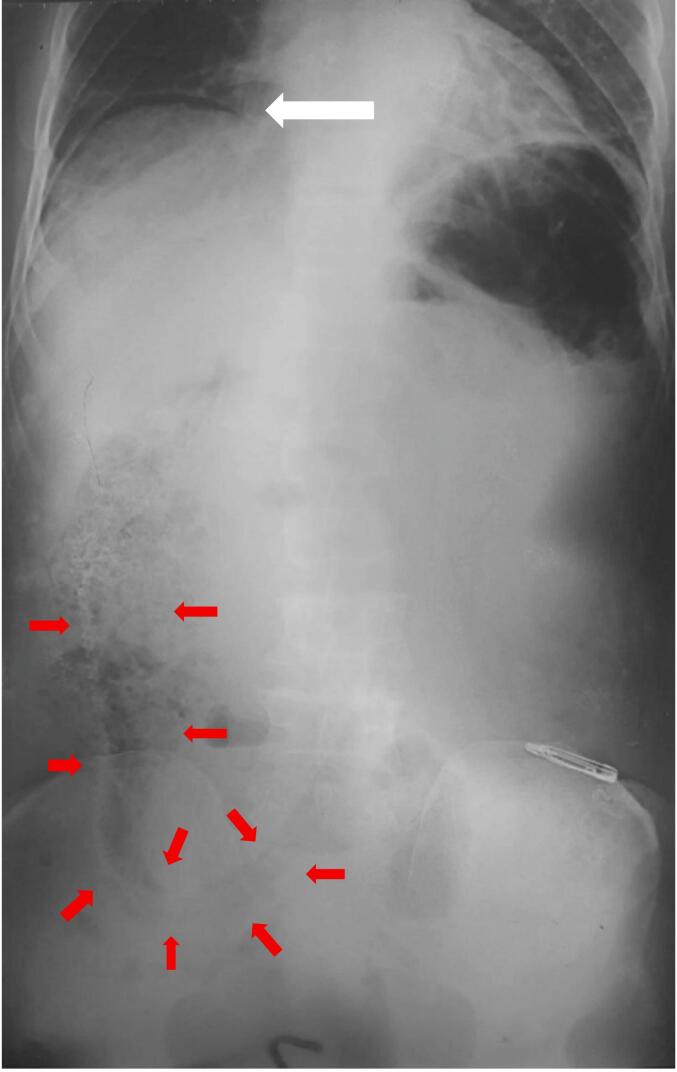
Plane X-ray abdomen in anterior-posterior view showing ground glass opacity and crescentic gas shadow under the right dome of diaphragm (white arrow). An obscure radiolucent shadow of the eel fish in right side of the abdomen. (The red arrow marks its course.) (For interpretation of the references to colour in this figure legend, the reader is referred to the web version of this article.)
